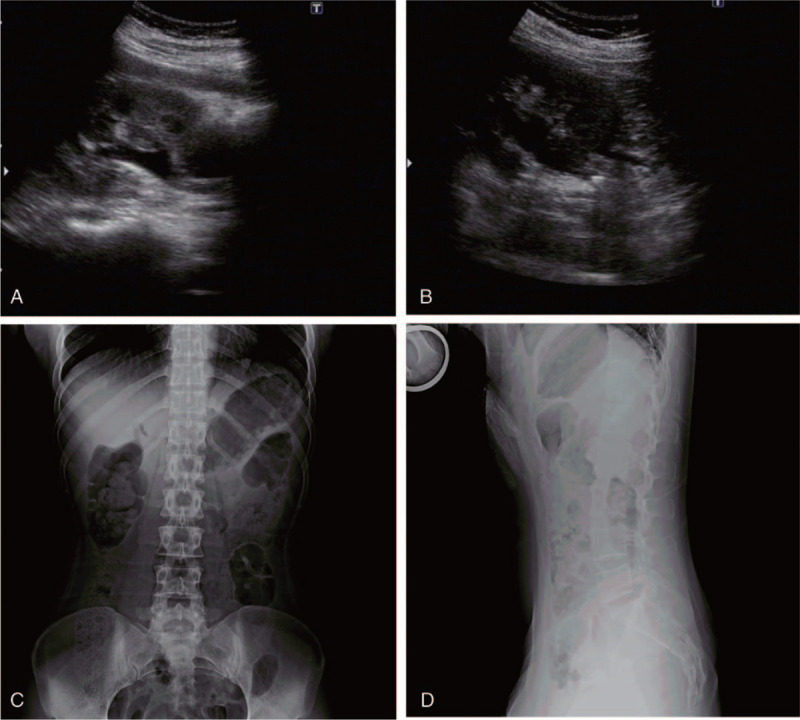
The urological Doppler ultrasound and radiograph of abdominal horizontal position radiograph (KUB) of patient 1. A and B, The urological Doppler ultrasound revealed hydronephrosis of the right kidney and right proximal ureteral calculus. C and D, The abdominal horizontal position radiograph in the right upper segment of the ureter deformable area showed high-density shadow because of the calculi.
